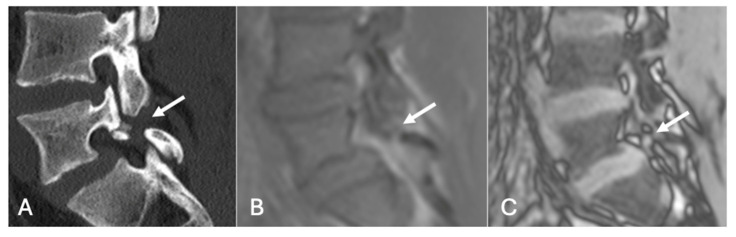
Pars defect assessment CT image (A) and chemical shift in-phase (B) and out-of-phase (C), showing pars defect of L5 (arrow).
